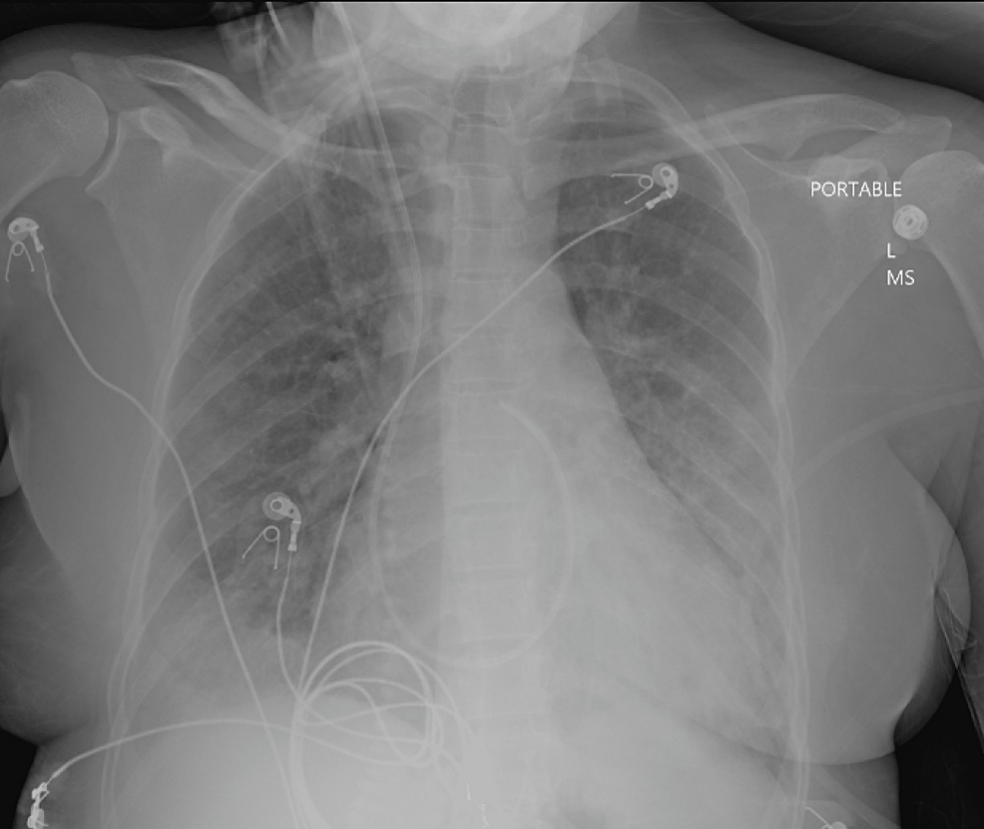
AP view of frontal chest X-ray depicting bilateral pulmonary edema and an enlarged cardiopulmonary silhouette.
